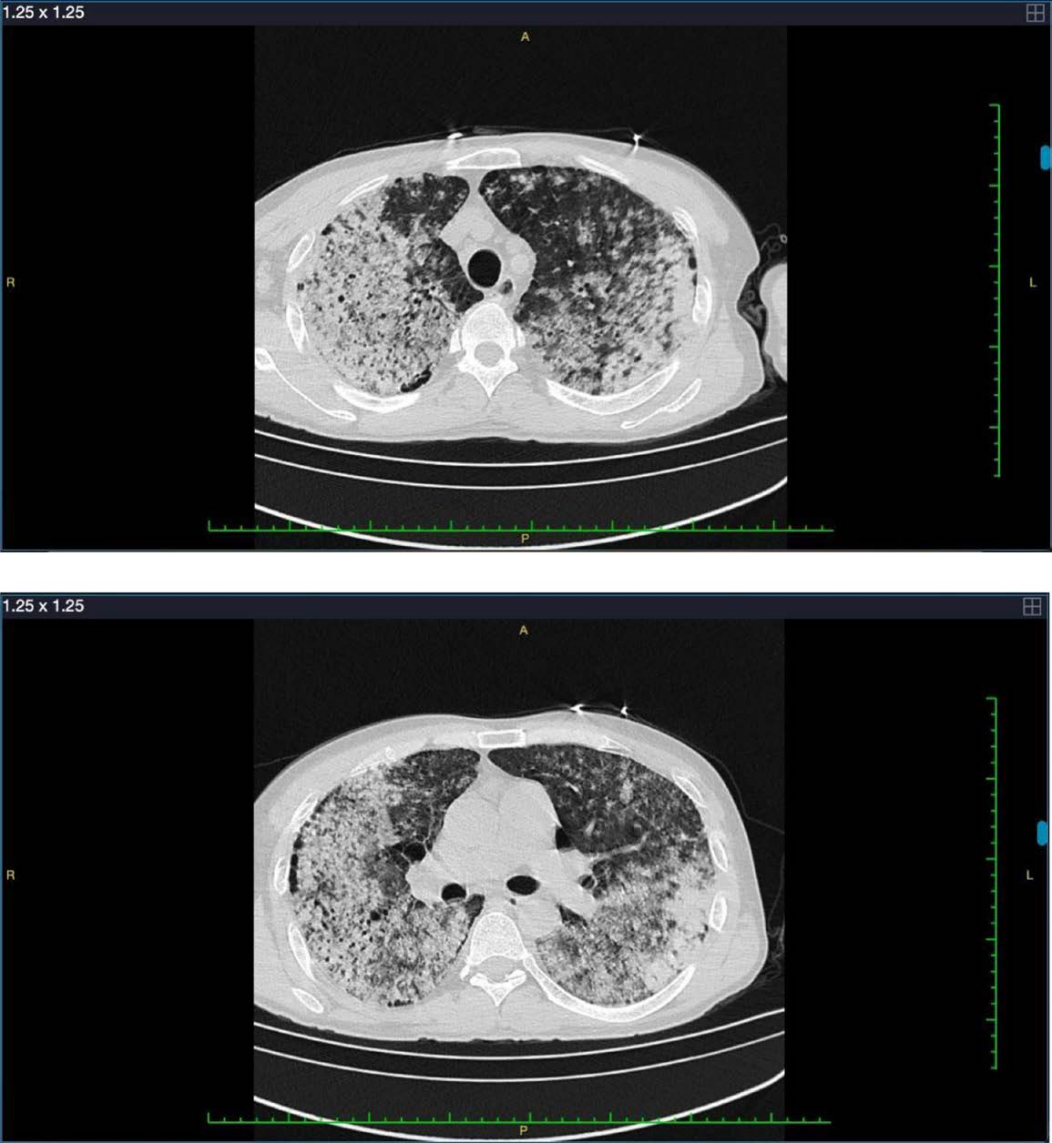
The chest computed tomography upon hospital admission on the first day of hospitalization showed diffusely increased-density.
